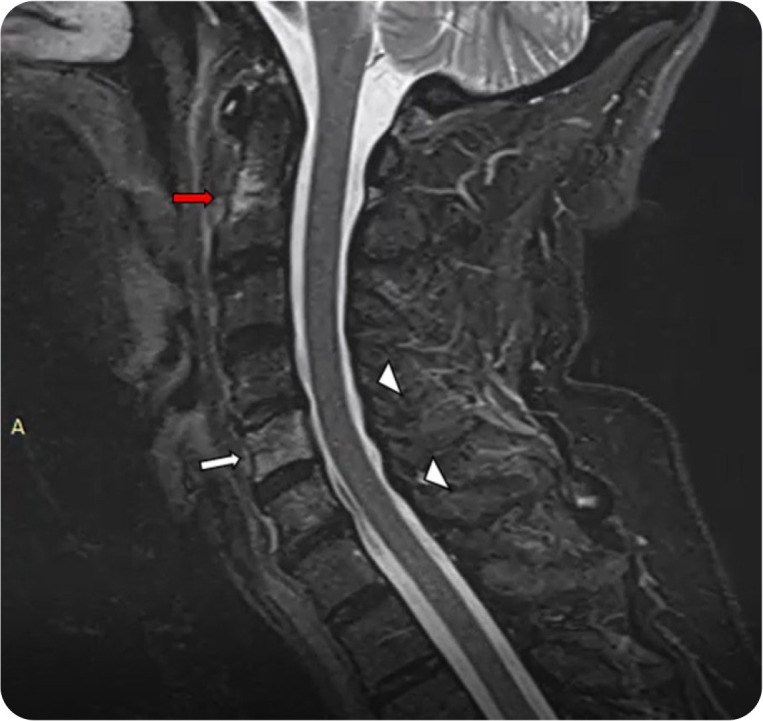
Magnetic resonance imaging STIR sequence: inflammatory spinal lesions- bone marrow oedema in C2 (red arrow) and extensive inflammation in C5 resembling osteitis (white arrow). Degenerative structural changes in C4-C5, C6-C7 (white arrowheads).
